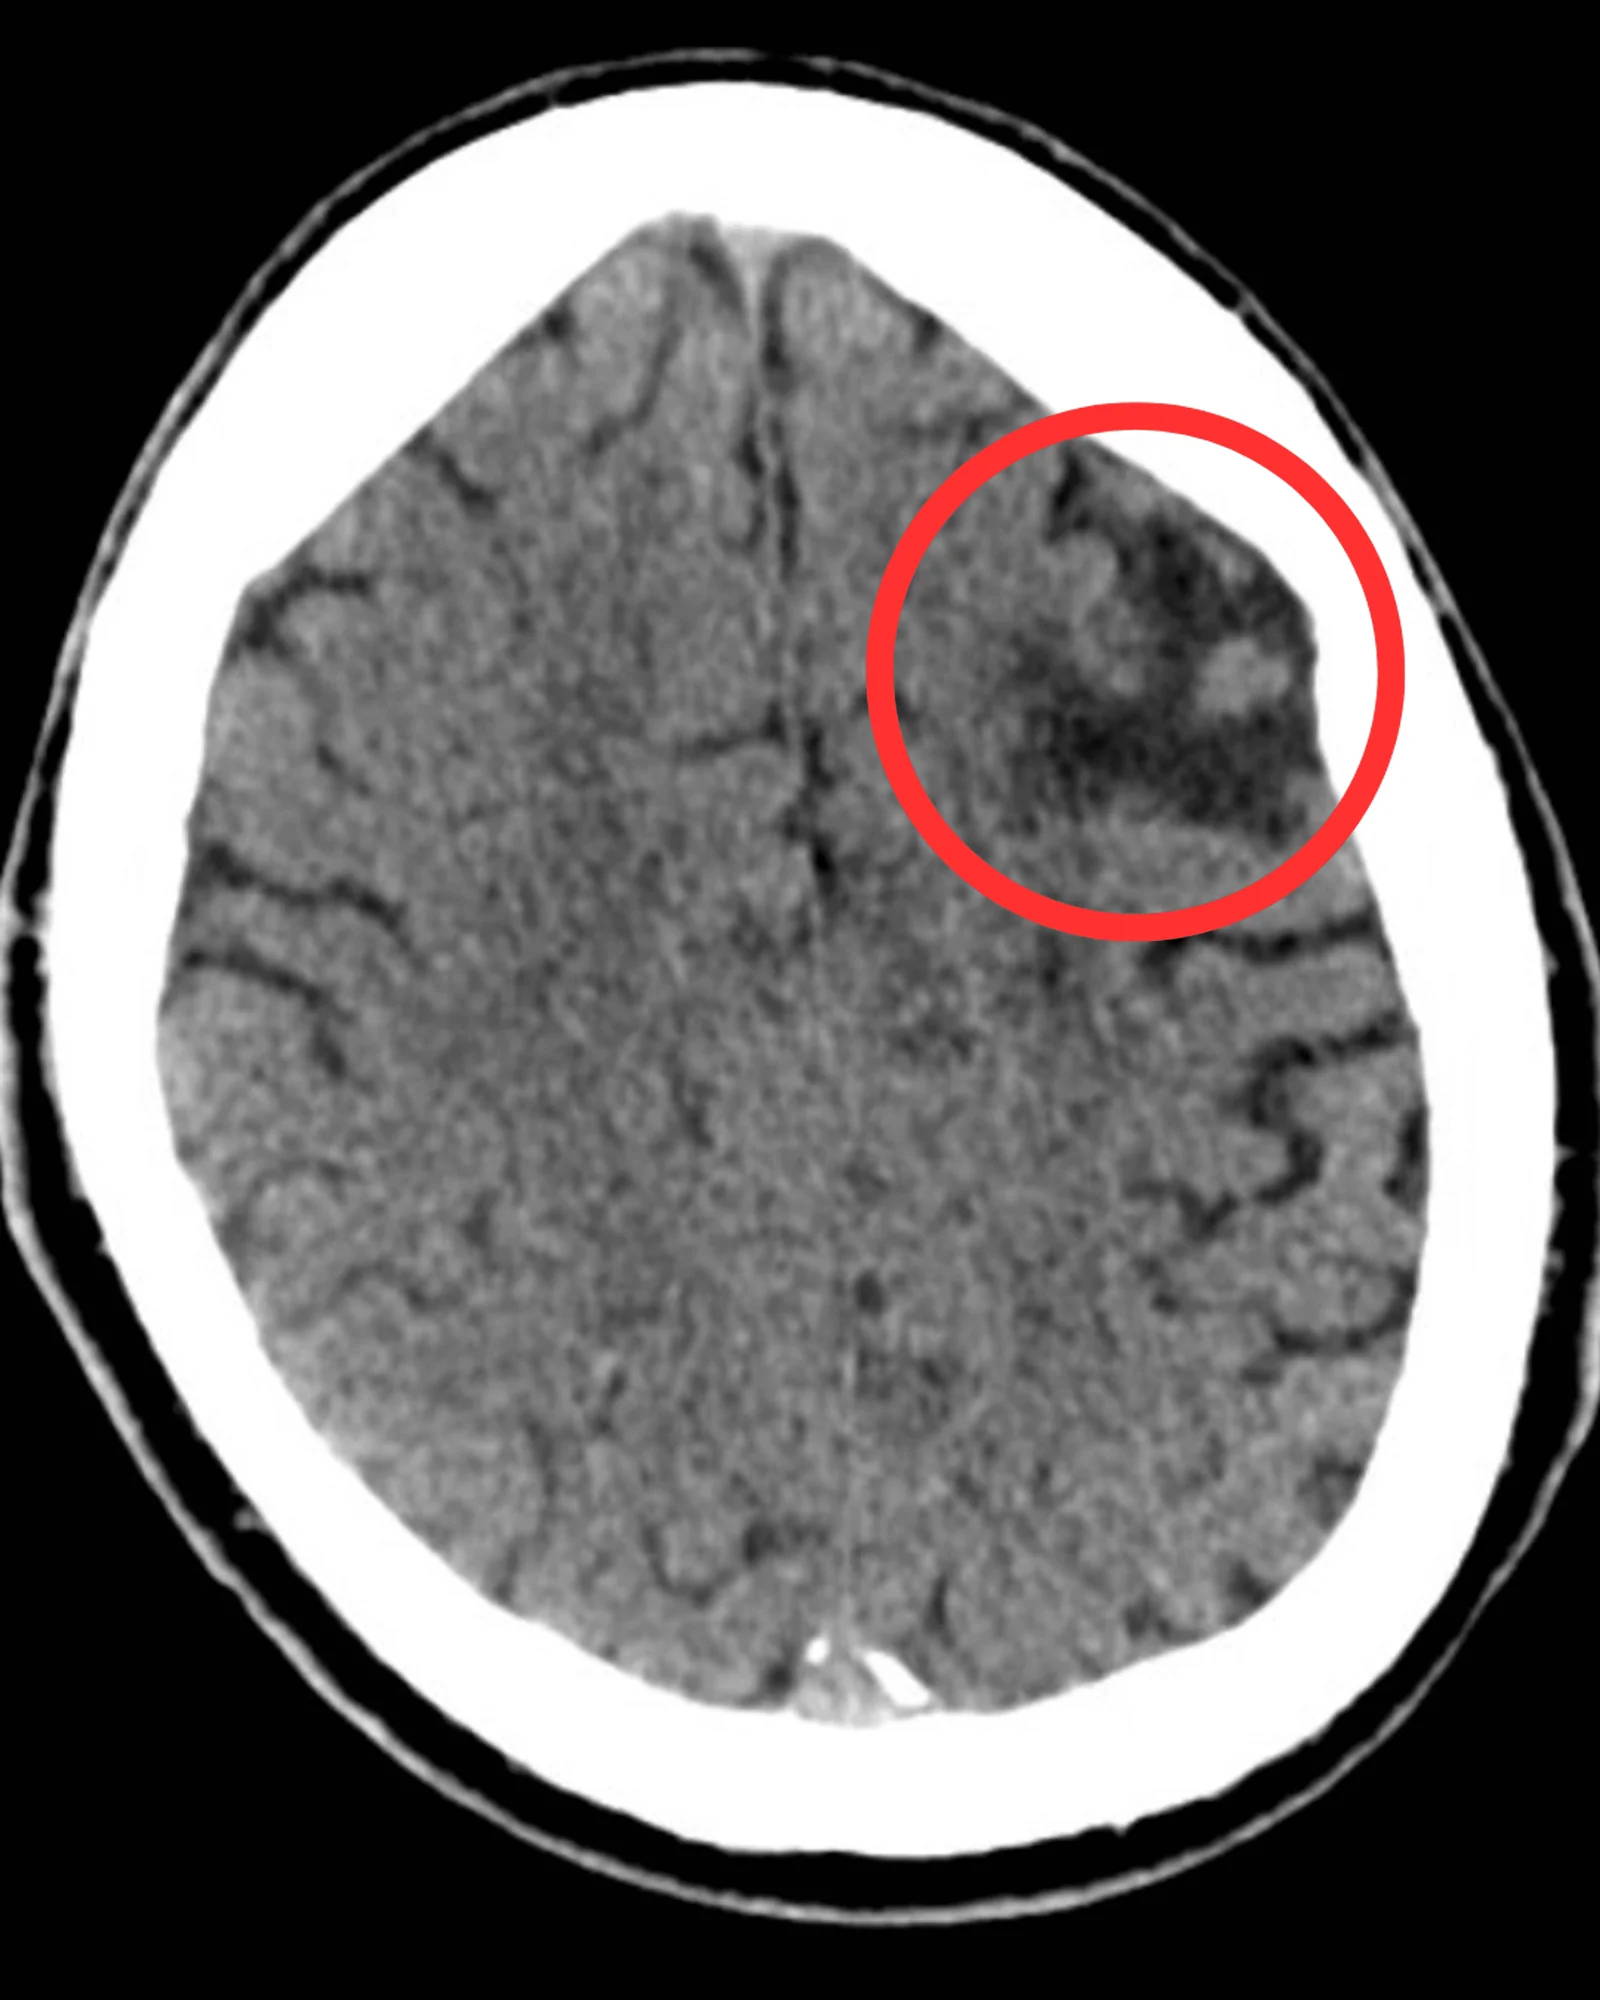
Brain CT on admission significant for stable areas of encephalomalacia in the left frontal and parietal lobes This finding delayed the diagnosis.
